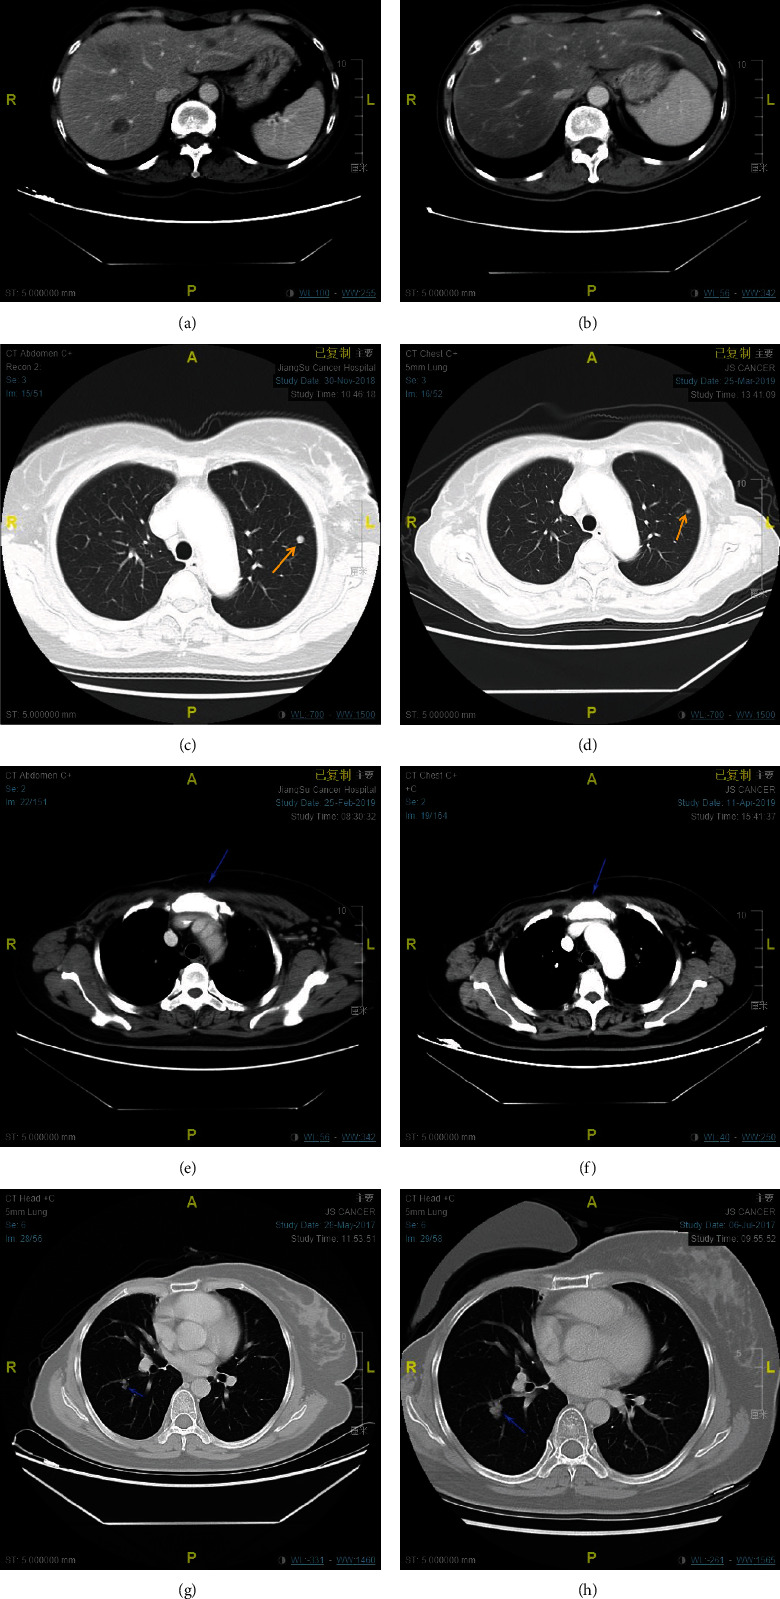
CT images of the patient before and after treatment. (a) CT images of patients with liver metastases diagnosed as CR before treatment; (b) CT images of patients with liver metastases diagnosed as CR after treatment with pyrotinib+capecitabine; (c) treatment of patients with pulmonary metastases diagnosed as PR CT images before treatment; (d) CT images of patients with pulmonary metastases diagnosed as PR after treatment with pyrotinib+capecitabine; (e) CT images of patients with pulmonary metastases diagnosed as SD before treatment; (f) CT images of patients with pulmonary metastases diagnosed as SD CT images of patients with pulmonary metastases treated with pyrotinib+capecitabine; (g) CT images of patients with pulmonary metastases diagnosed as PD before treatment; (h) CT images of patients with pulmonary metastases diagnosed as PD treated with pyrotinib+capecitabine CT images after the treatment.
